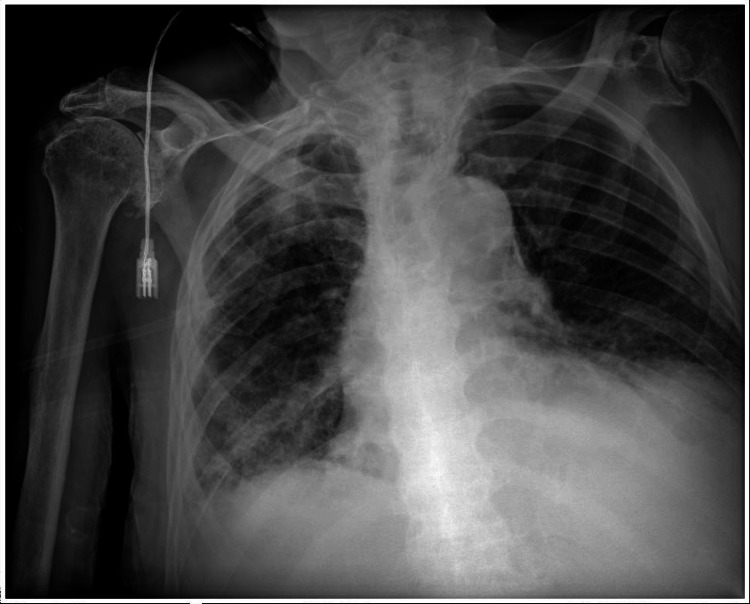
Chest radiography Areas of hypotransparency in the bilateral basal and left retrocardiac regions are consistent with inflammatory-infectious etiology, along with a bilateral basal pleural effusion.
